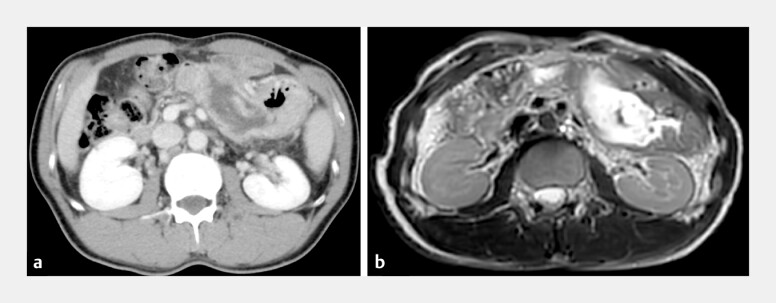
Computed tomography (CT) and magnetic resonance imaging (MRI) of gastric penetration by intraductal papillary mucinous neoplasms (IPMNs). Contrast-enhanced CT (CE-CT) revealed a markedly dilated main pancreatic duct (MPD) and thin pancreatic tissue.
